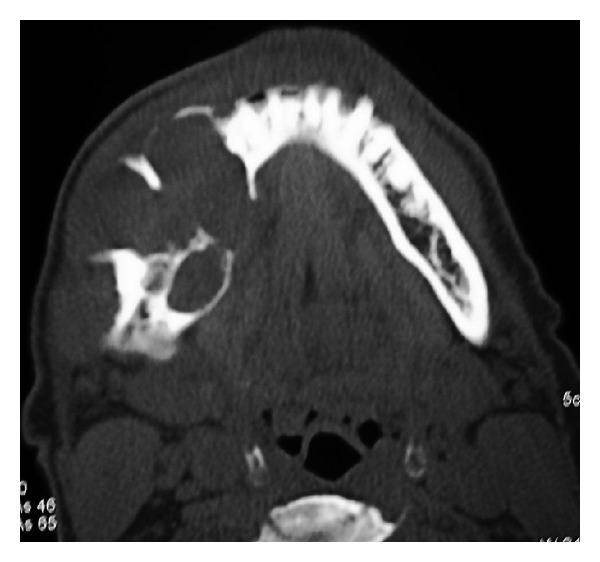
Axial view CT scan showing bicortical expansion with perforation of the buccal and lingual cortices on the right side.
